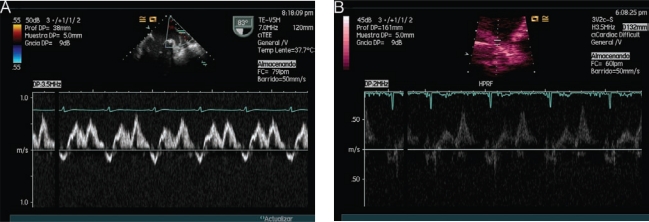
Diastolic function study: Pulsed Doppler in pulmonary veins. A) TEE image, B) TTE image. In both, systolic wave < diastolic wave.
